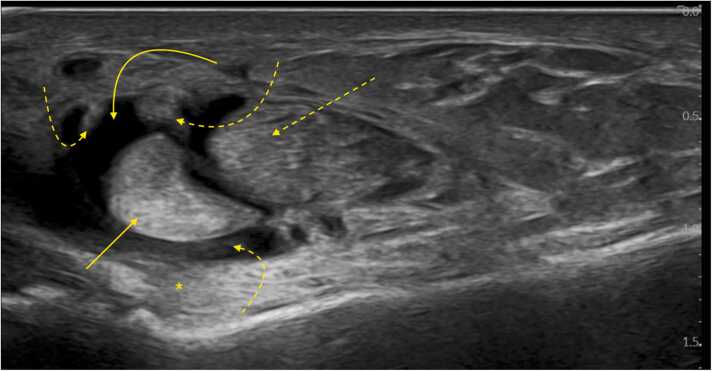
A patient with pain at and below the level of the lateral malleolus. Ultrasound, oblique section just below the level of the lateral malleolus, shows effusion in the common peroneal synovial sheath (curved arrow) and thickening of the synovial membrane (curved dashed arrows). The peroneus brevis (straight arrow) and peroneus longus (straight dashed arrow) tendons are intact but exhibit degenerative changes and moderate swelling. The calcaneofibular ligament is marked with an asterisk.
